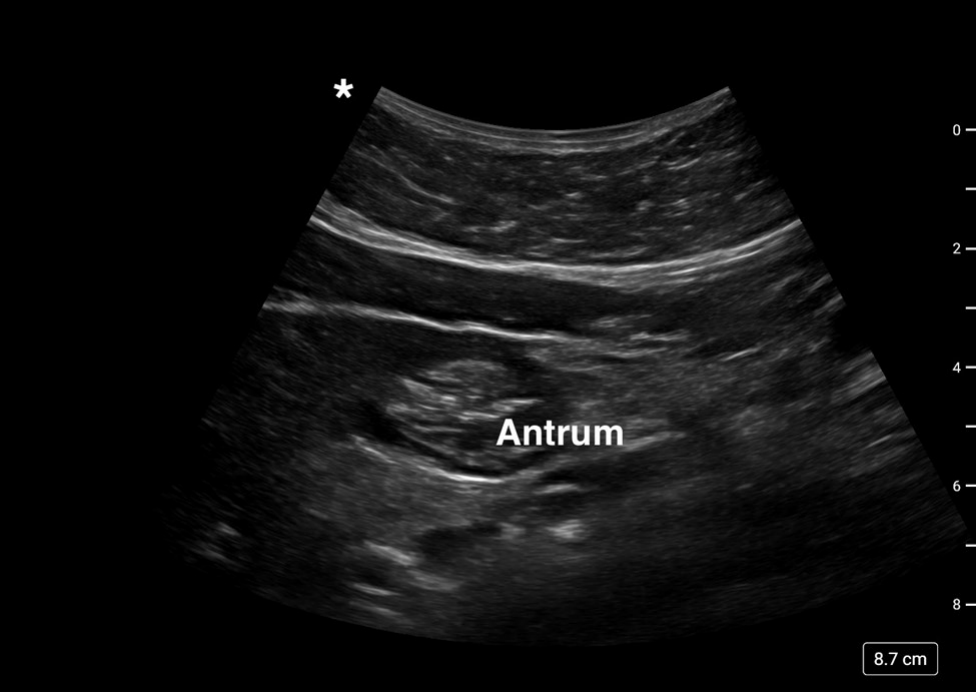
Gastric ultrasound image case #3
